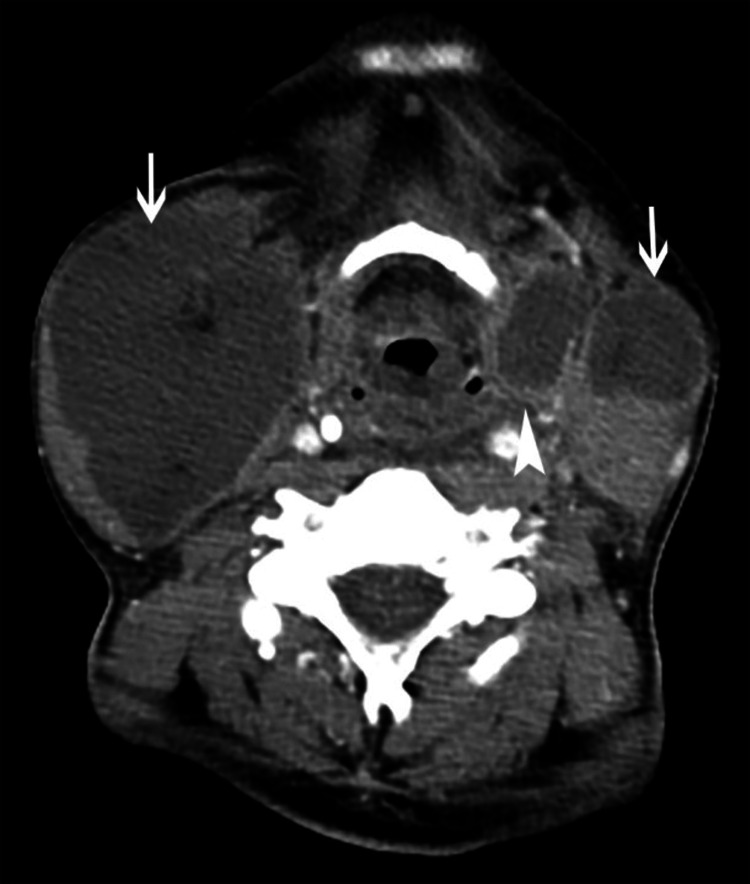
CT scan of the neck showing extensive myonecrosis involving bilateral sternocleidomastoid muscles (arrows). There is a paralaryngeal abscess (arrowhead) extending beyond thyrohyoid membrane in larynx.
